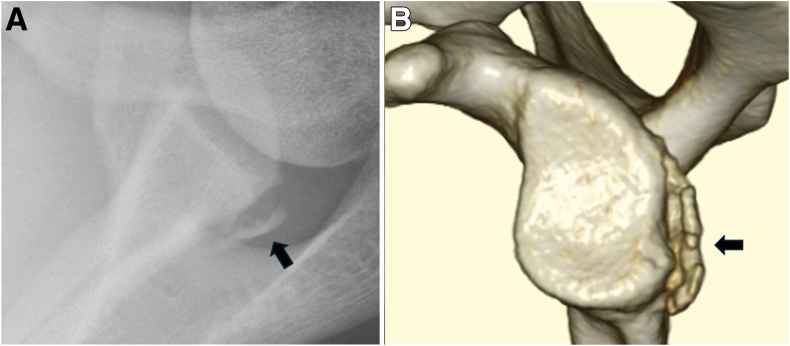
Image finding of the posterior bony Bankart lesion in the left shoulder. Arrows indicate the lesion. (A) Radiograph: Axillar view shows a posterior bony Bankart lesion. (B) Three-dimensional computed tomography view.
